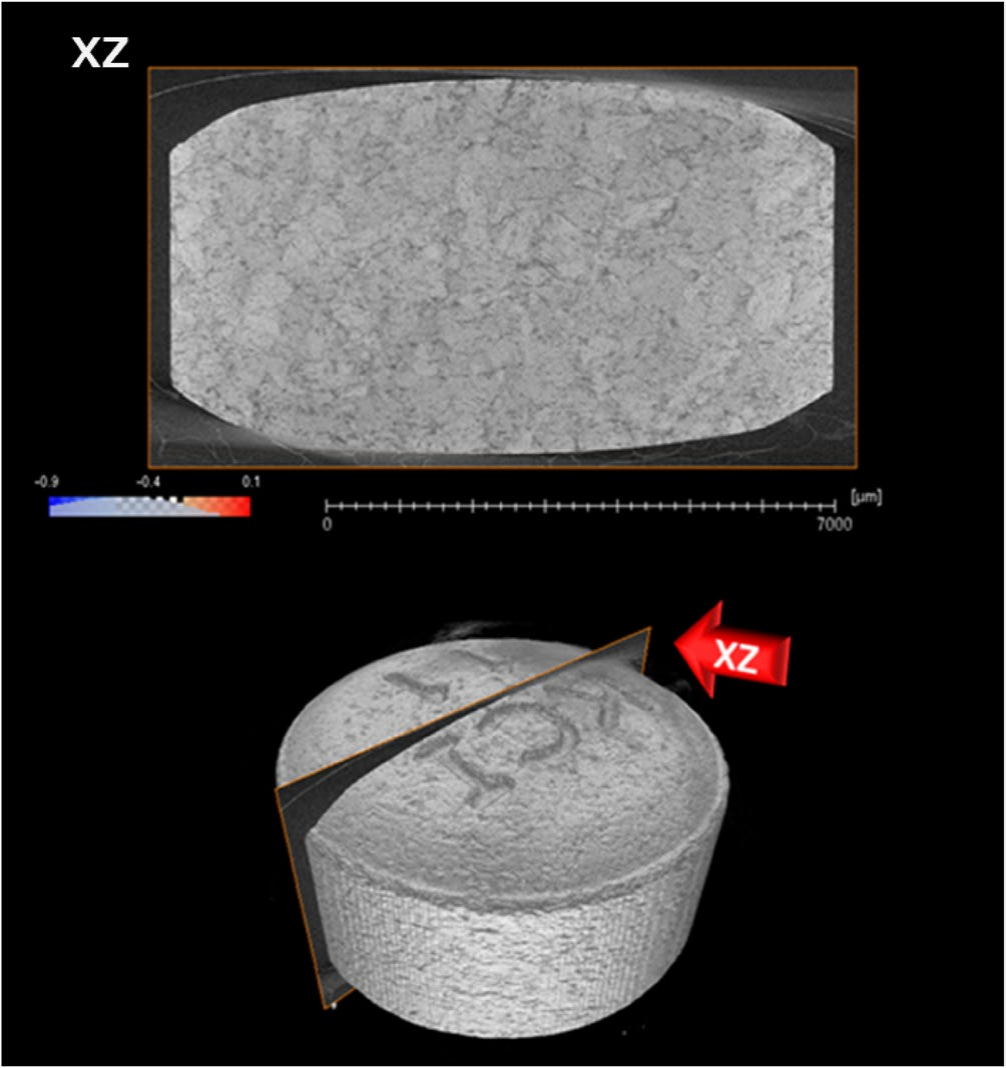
X-ray CT data of the MgO tablet. X-ray radiography of the sample was performed using a ZEISS Xradia 520 Versa (ZEISS) with a voltage of 60 kV and a power of 5.0 W. X-ray CT data were computed from the obtained X-ray radiographs. The virtual slice and a three-dimensional representation are presented.
